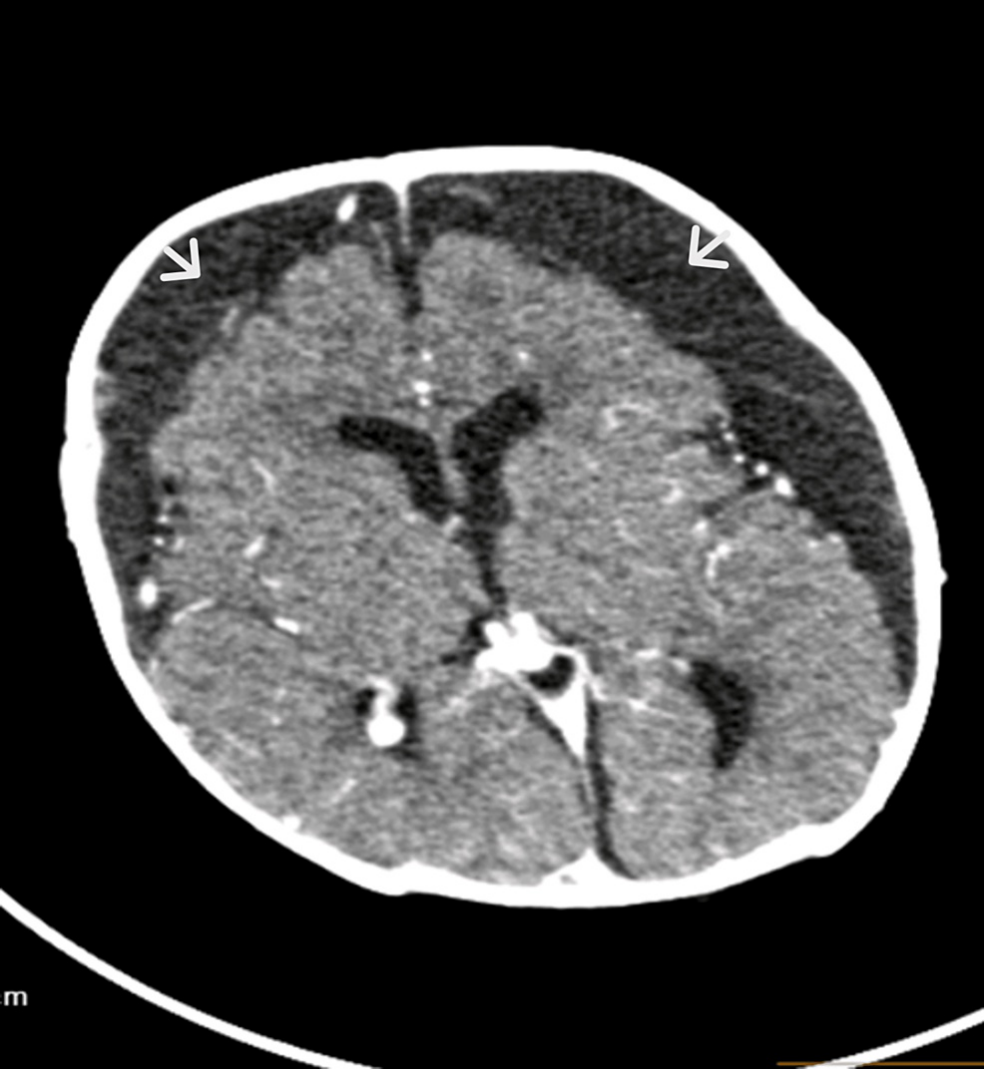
Repeat CT scan of the brain shows an interval increase in right subdural collections that measures 1.7 cm compared to the previous measurement of 1.1 cm (white arrows)
